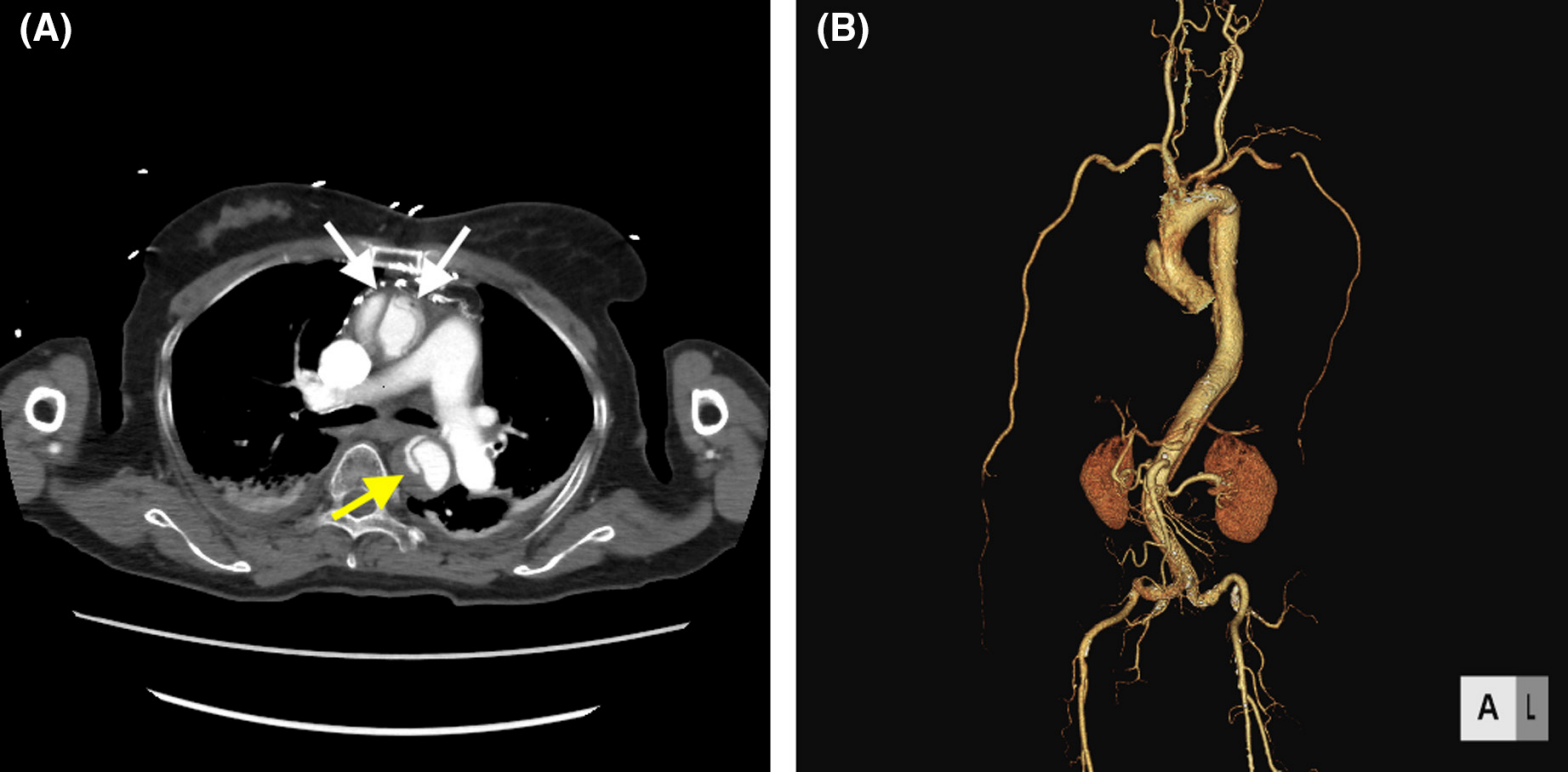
(A) Computed tomography imaging showing the new intimal flaps at ascending aorta (white arrow) and descending aorta (yellow arrow). (B) 3D computed tomography imaging showing the dissection progressed from ascending aorta to the terminal aorta.
